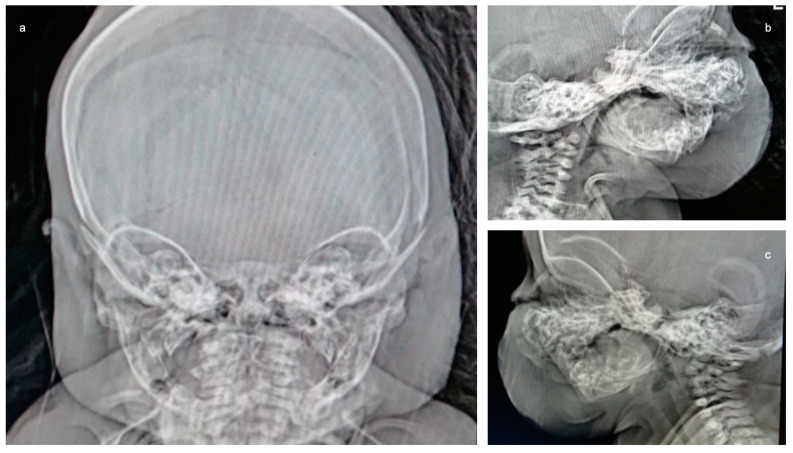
X-ray of the skull: On both the AP image and the lateral projections, it is difficult to recognize the duplication of the maxilla and mandible. The coronal and sagittal sutures are wide.
